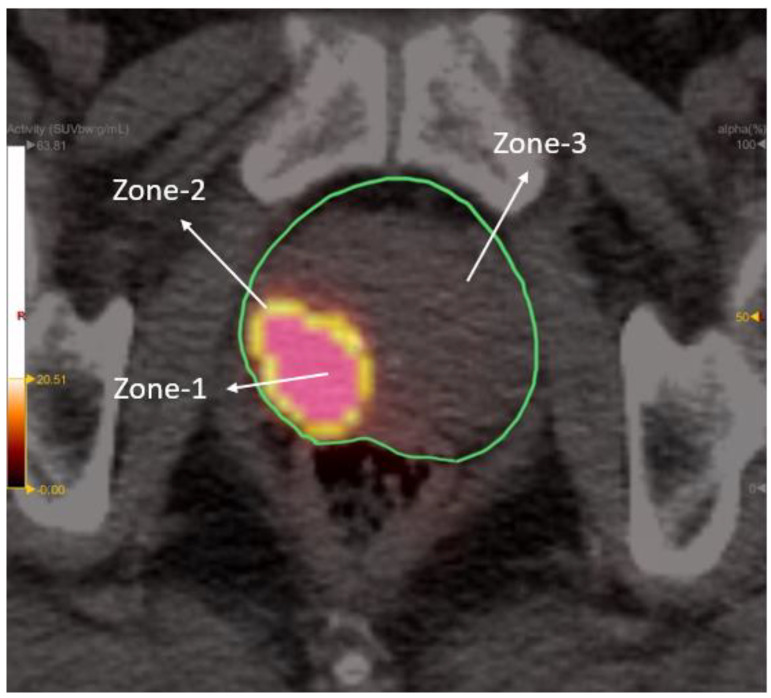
Depiction of the three radiomic zones on a PET/CT image. Standardized uptake values for zone-1 and zone-2 were 45–100% and 20–45% of maximum standard uptake value (SUVmax), respectively. Zone-3 comprised the entire prostate with the exclusion of zone-1.
